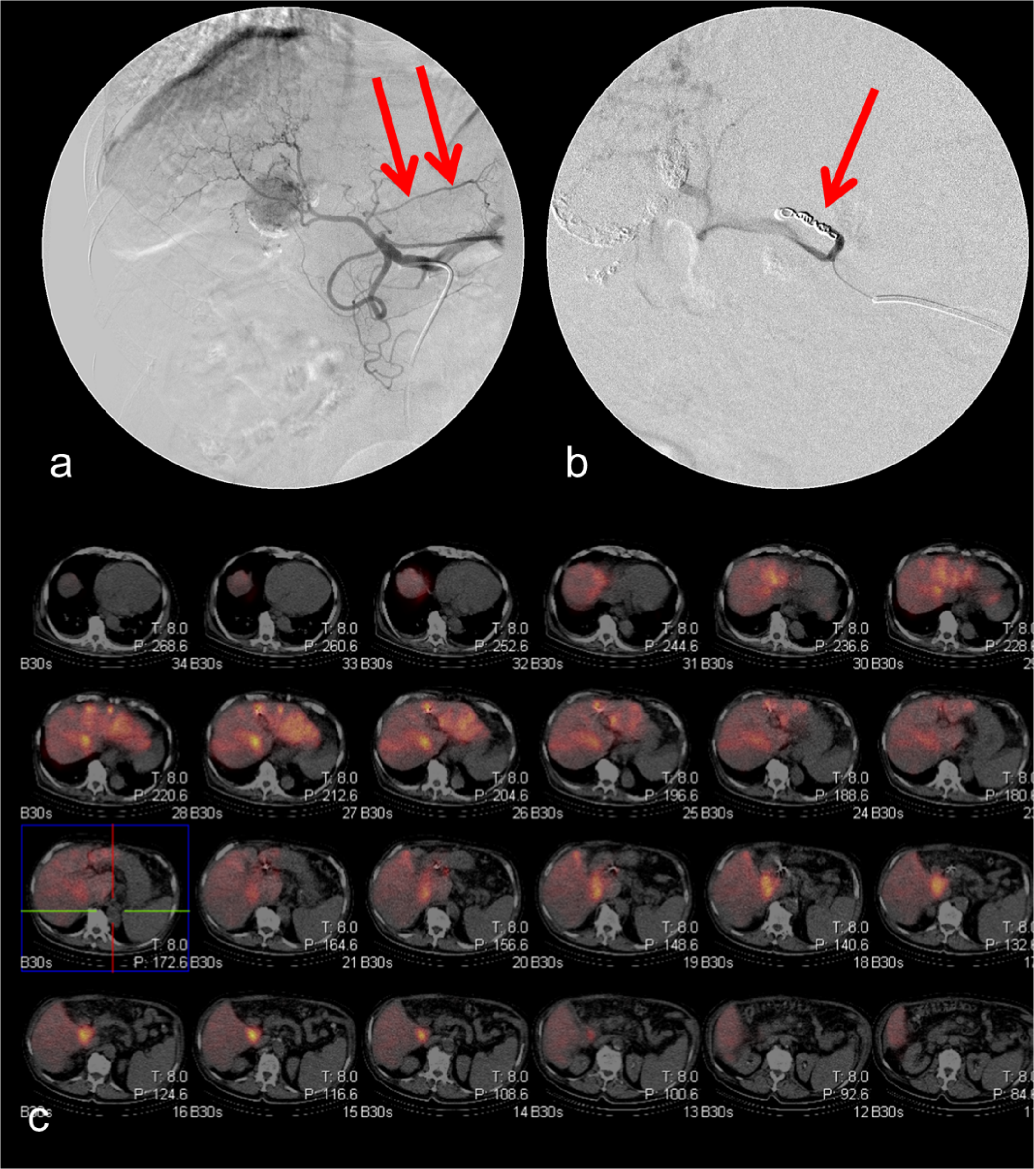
65 year-old male patient. The right gastric artery (Fig 1a; arrow) was identified and coilembolized (Fig 1b). SPECT/CT (Fig 1c) did not reveal extrahepatic tracer deposition.
